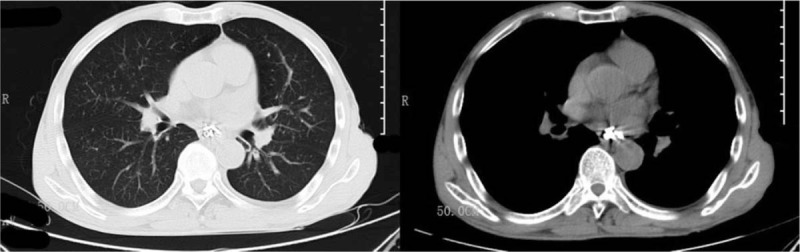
Recheck chest CT was showed mediastinal emphysema and subcutaneous emphysema disappeared 17 days after the operation. CT = computed tomography.
